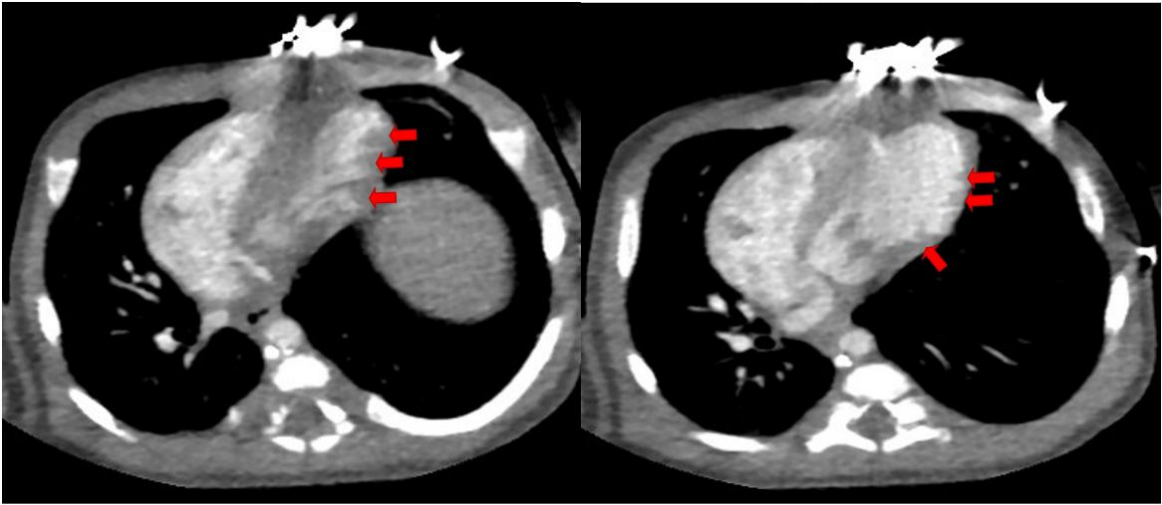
Cardiac computed tomography angiography. *(A)* A saw-tooth pattern is observed during the systolic phase (indicated by arrows). *(B)* During the diastolic phase, projections resembling a saw-tooth pattern (also indicated by arrows) can be seen.
